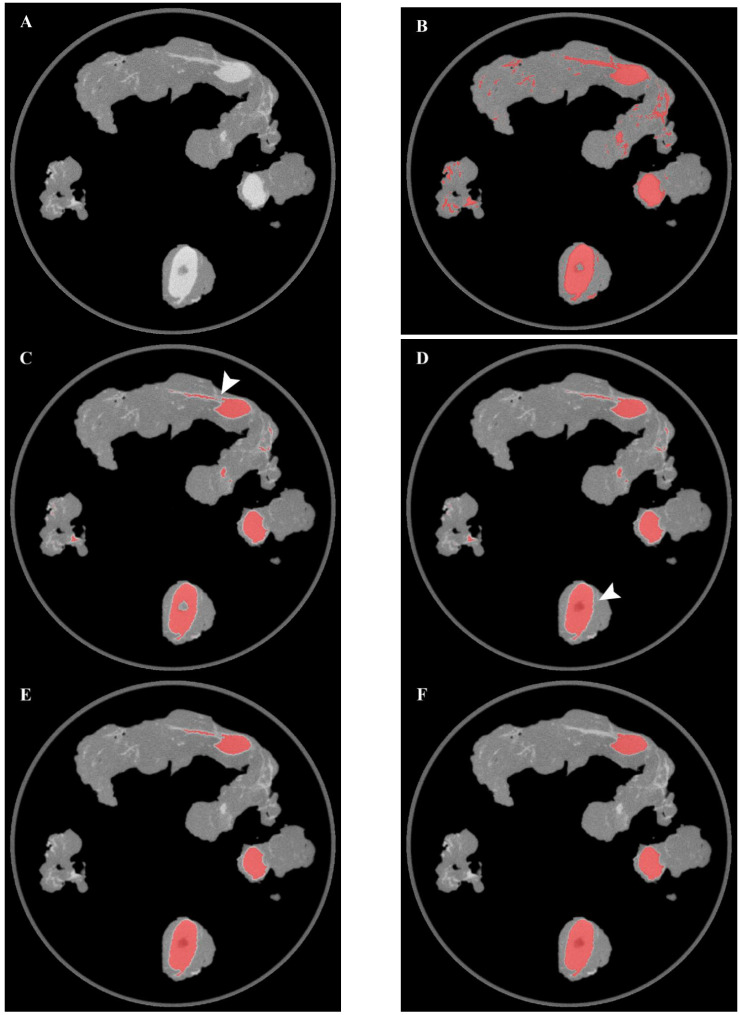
Overview of the results of the main segmentation steps on a sample axial image. (A) original CT image, (B) K-means clustering, (C) Erosion, (D) Filling, (E) 2D noise removal, (F) 3D noise removal. The erosion process allowed to detach two wrongly connected objects (arrow in panel (C)), while the hole-filling operation allowed to include the lymph node hilum in the segmented area (arrow in panel (D)).
